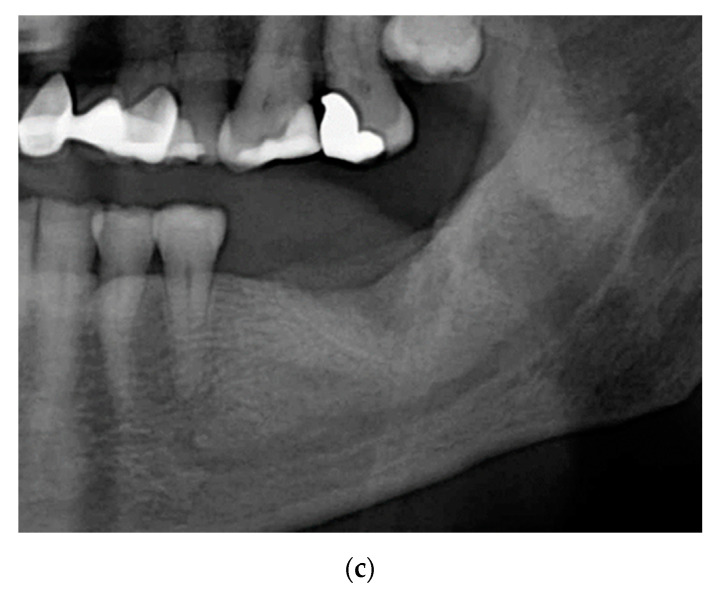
(a) Affected area 15 days post-surgery, (b) 30 days post-surgery and (c) X-ray 90 days post-surgery.
